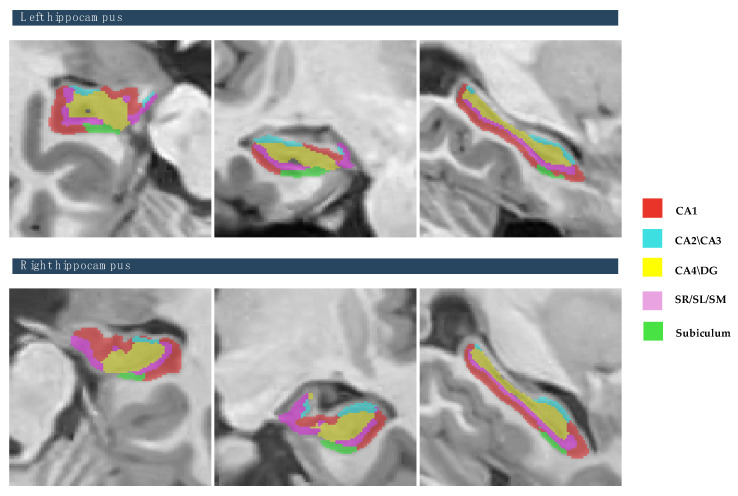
The process of hippocampal subfields segmentation based on the Winterburn atlas. Right panel shows the color coded of hippocampal subfields that are overlaid onto the subject’s high-resolution T1-weighted anatomical scan [subject 10].
